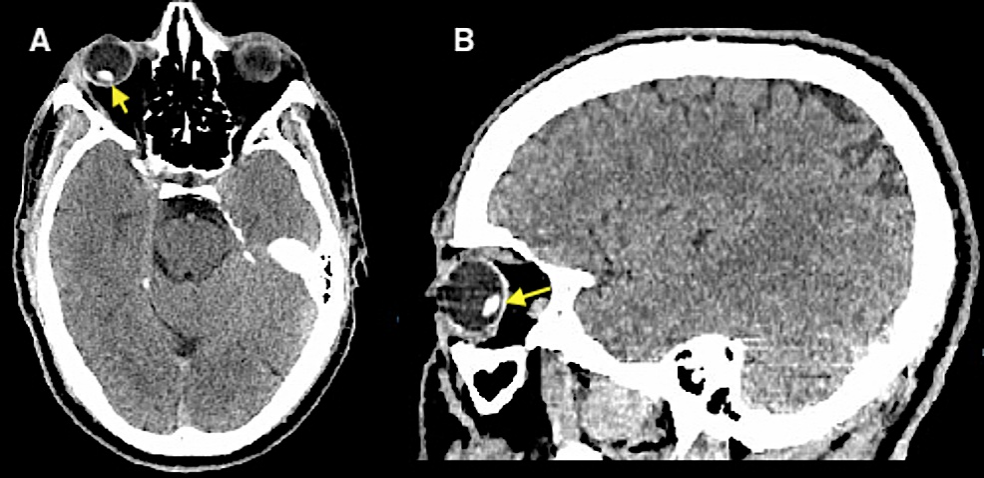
CT demonstrating a posterior lens dislocation of right orbit. A: Axial plane; B: Sagittal plane; Dislocated lens (yellow arrows).
